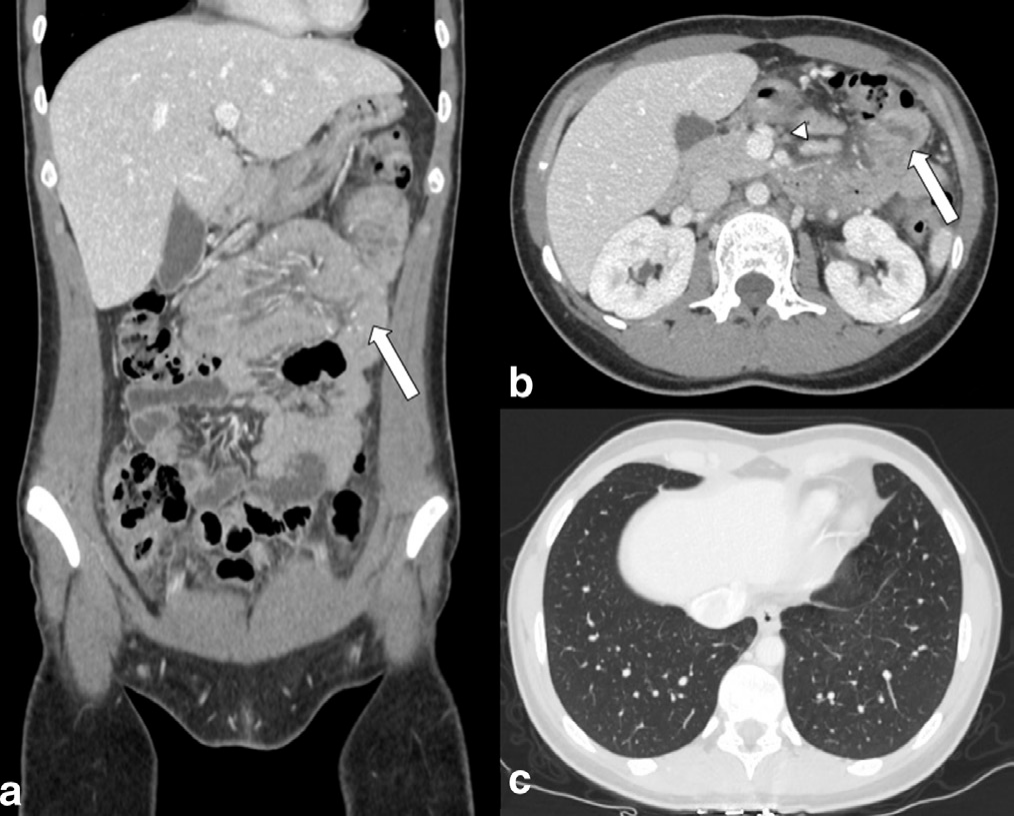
Enhanced CT scan (portal phase) of the abdomen of a 25-yo female admitted to
